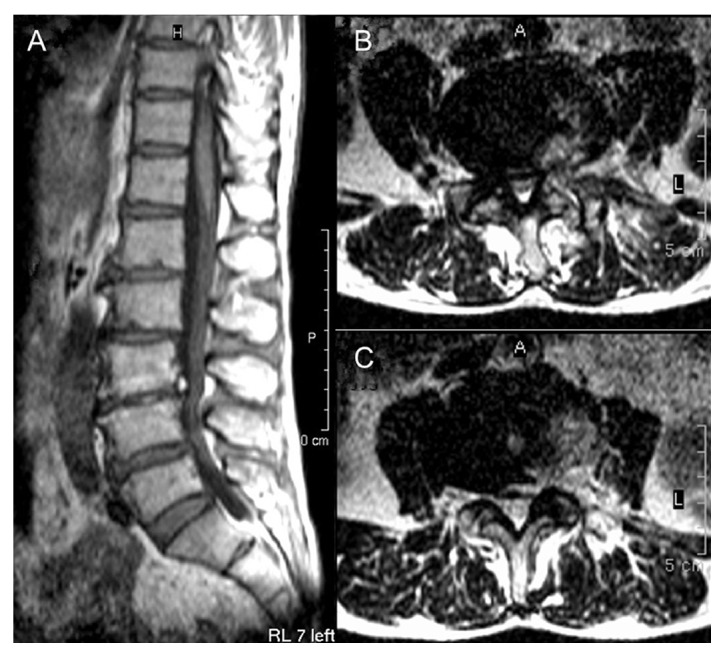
Both the T1 sagittal (A) and coronal MRI images of L3-4 (B) and L4-5 (C) at 1-month postoperatively, indicating that the herniation and stenosis at both levels were alleviated compared with the preoperative results, despite edema being observed.
